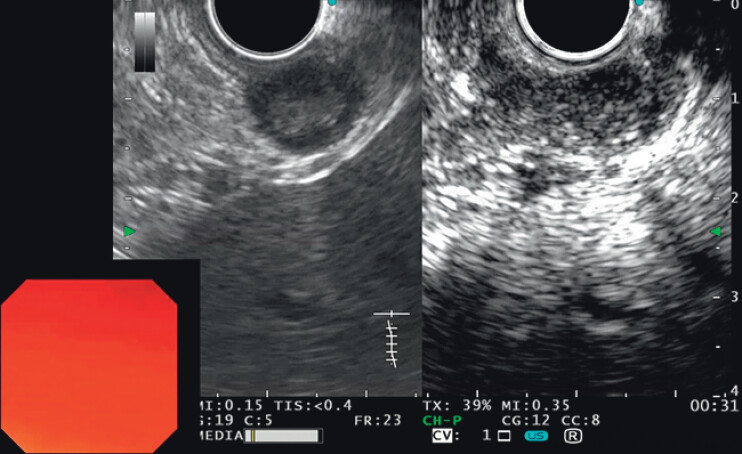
EUS showed internal hyperechoic foci; CEH-EUS revealed early and heterogeneous enhancement. EUS, endoscopic ultrasound; CEH-EUS, Contrast-enhanced harmonic EUS.
